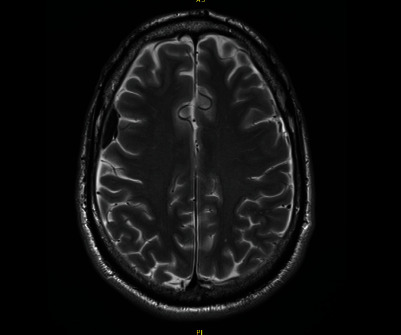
MRI showing left SDH taken at time of presentation in the emergency department.
